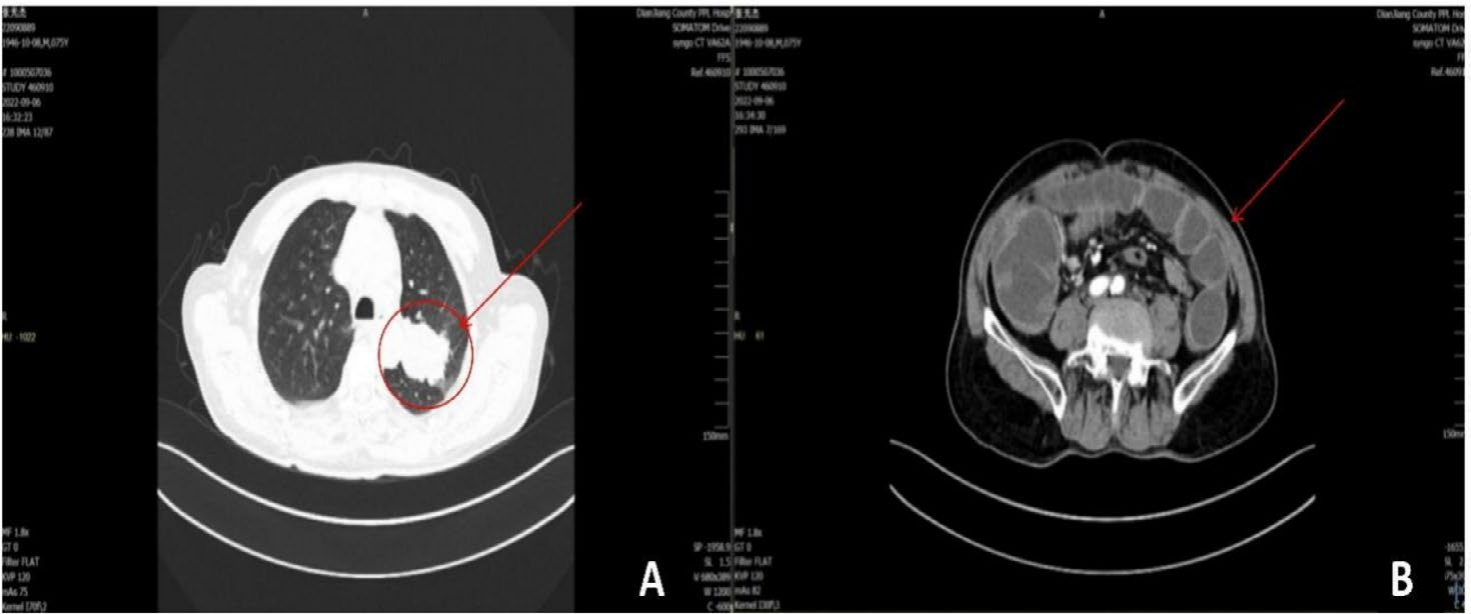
Imaging examination: Contrast‐enhanced chest CT shows a mass in the left upper lobe (5.8 cm × 4.2 cm), suggesting progression of the patient's primary lung cancer (Figure 1A). Contrast‐enhanced abdominal CT shows intestinal volvulus with a “vortex sign,” supporting the radiological diagnosis of intestinal obstruction (Figure 1B).
